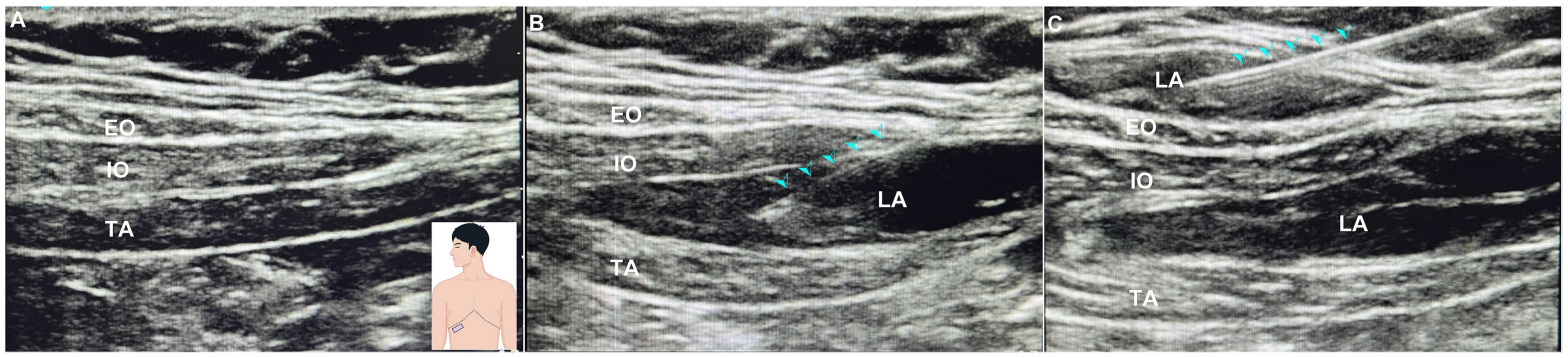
The novel ultrasound-guided transversus abdominis plane block approach. (A) Pre-block ultrasound probe placement and ultrasound images; (B) the initial injection: needle-tip placement at the fascia between the internal oblique and transversus abdominis as visualized using ultrasonographic imaging; (C) the second injection: needle-tip placement at the superficial fascia of the external oblique muscle as visualized using ultrasonographic imaging.
